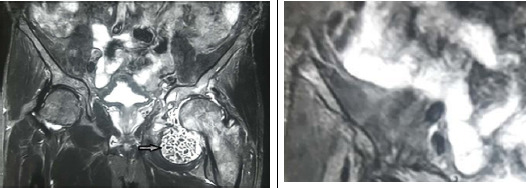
X-ray showing preoperative magnetic resonance imaging of hip joints showing in numerous loose bodies as shown by arrow suggestive of synovial chondromatosis.
